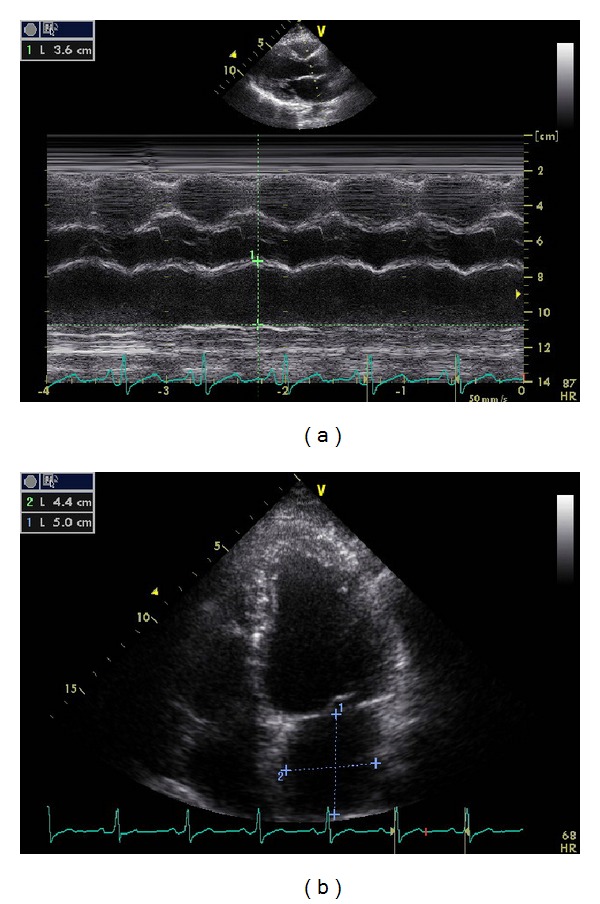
LA dimensions: anteroposterior diameter in parasternal long-axis view (a); longitudinal and transverse diameters in 4-chamber view (b).
